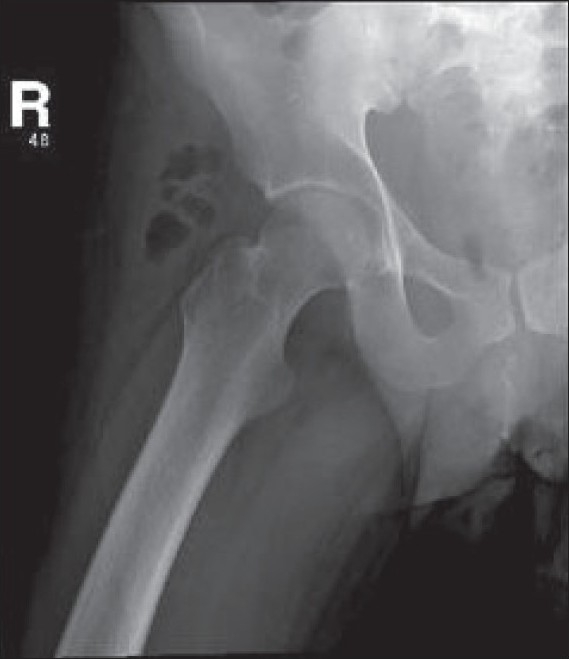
Plain X-ray of the right hip and thigh X-rays
